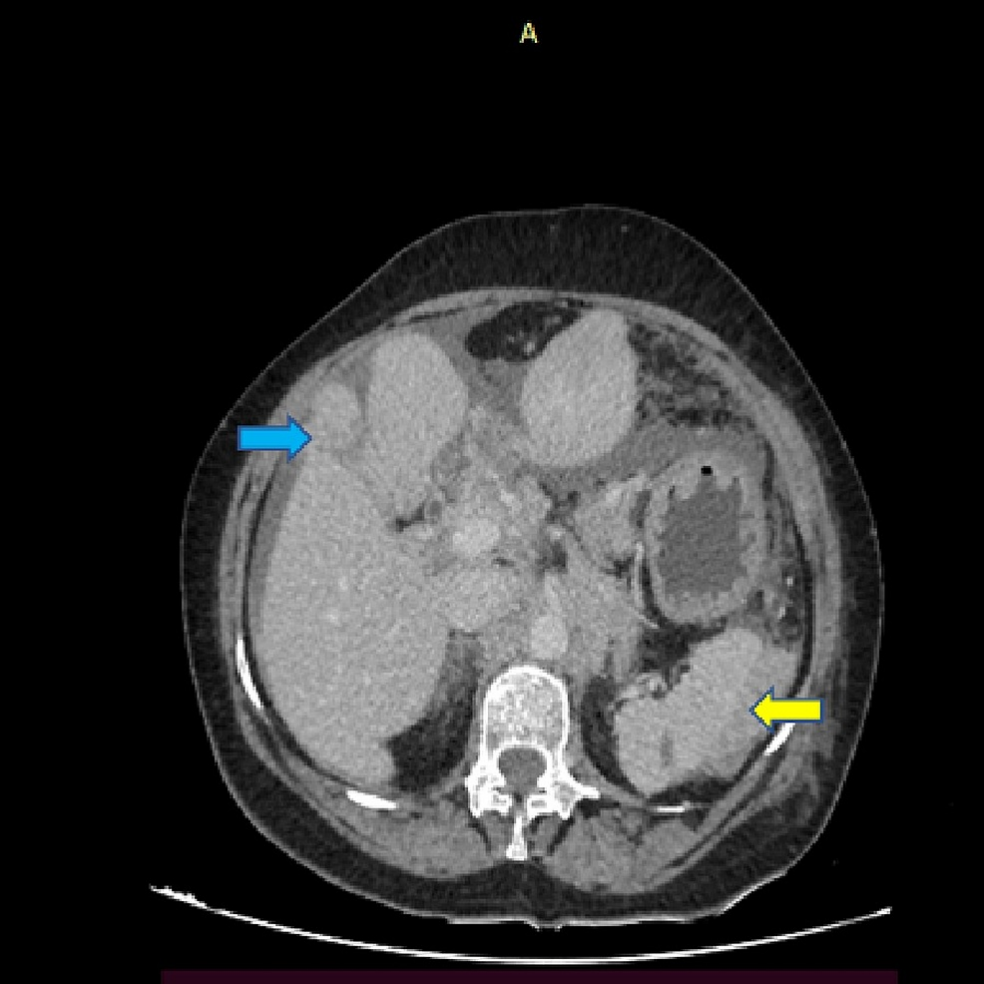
Image of axial section of CECT abdomen showing surface deposits over liver parenchyma (blue arrow) and spleen (yellow arrow) CECT - contrast-enhanced computed tomography
